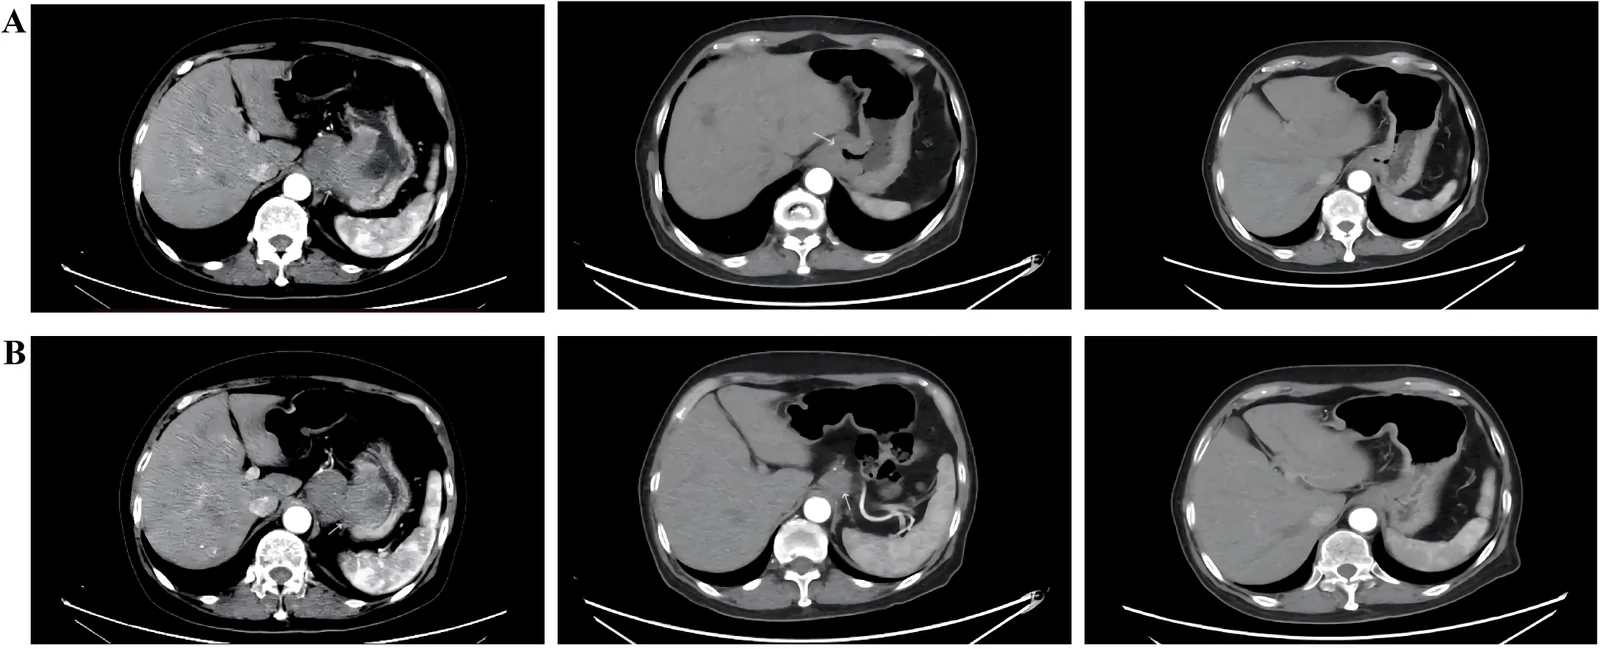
Computed tomography (CT) showed from the right to the left: Jan.2023, Apr. 2023, Jun.2023. (A) The wall thickness of the cardia and the bottom of the stomach were seen as follows: 5.42cm→3.84cm→2.54cm, it is significantly smaller than before. (B) There were many enlarged lymph nodes around the stomach, and the short diameter of the large ones almost disappeared by 1.5cm”.
